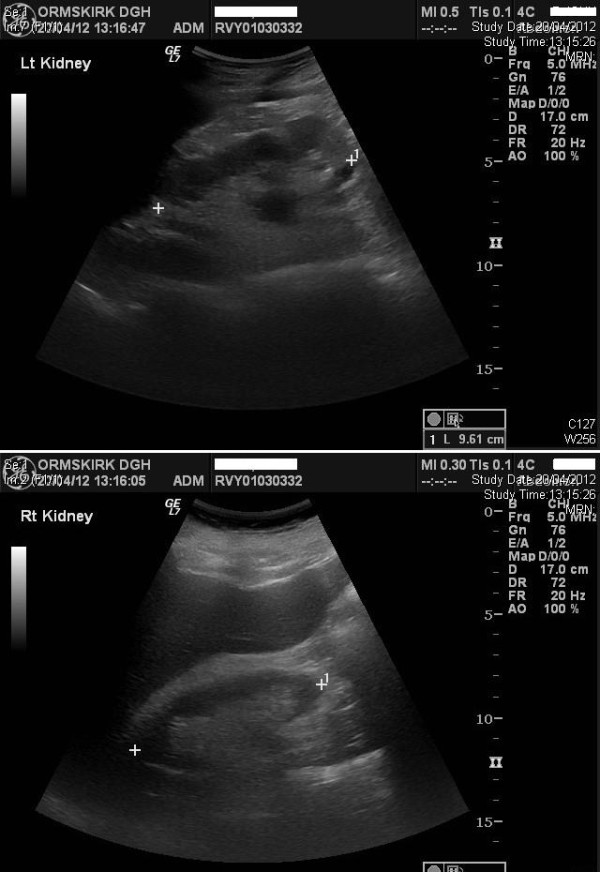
A (Top panel): Ultrasound examination, performed five months after establishing indwelling urethral catheter drainage, revealed normal right kidney with no evidence of hydronephrosis. B (Bottom panel) Ultrasound examination, performed five months after establishing indwelling urethral catheter drainage, revealed normal left kidney with no evidence of hydronephrosis.
